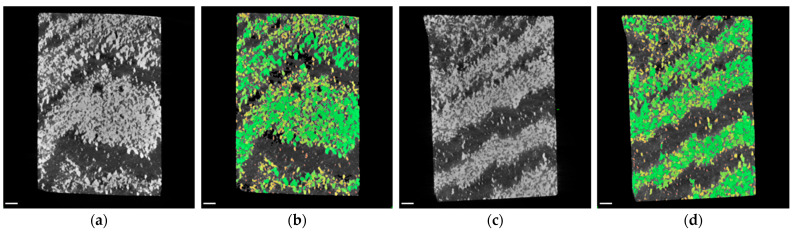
CT examination of banded iron ores: (a) virtual cross section through reconstruction in XZ‑plane of sample I; (b) results of blob analysis of sample I, identified blobs are labeled green, orange and yellow (colors are related to blob size); (c) virtual cross section through reconstruction in XZ‑plane of sample II; (d) results of blob analysis of sample II. Scale bars are 2 mm.
